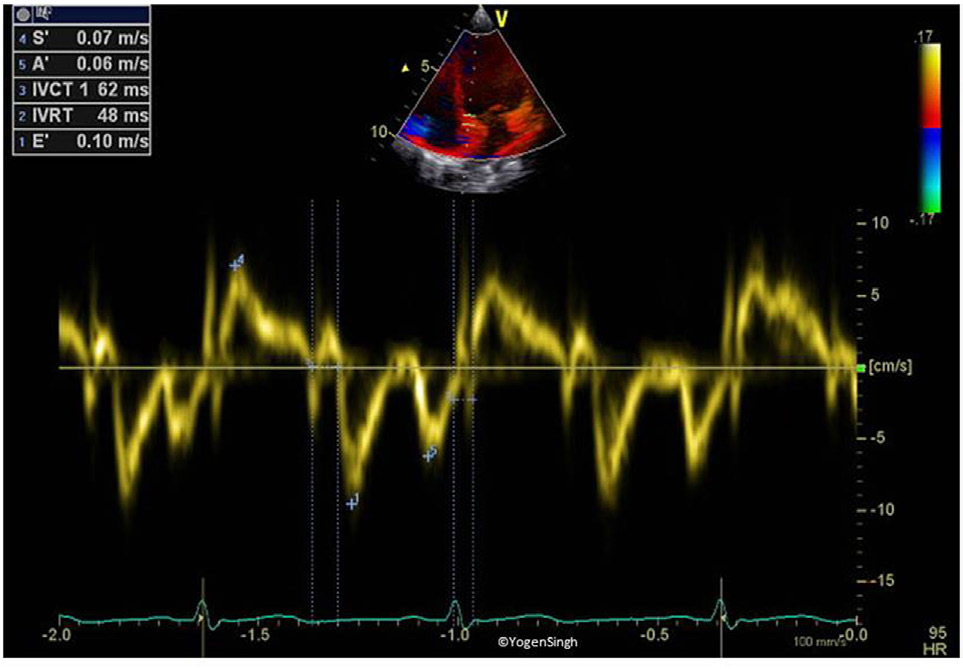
Tissue Doppler imaging on pulse-wave Doppler of interventricular septum (myocardium). Spectral above the baseline (s′) reflects the movement of myocardium toward apex in systole while below the baseline (e′ and a′) reflects the movement of myocardium away from apex in diastole.
